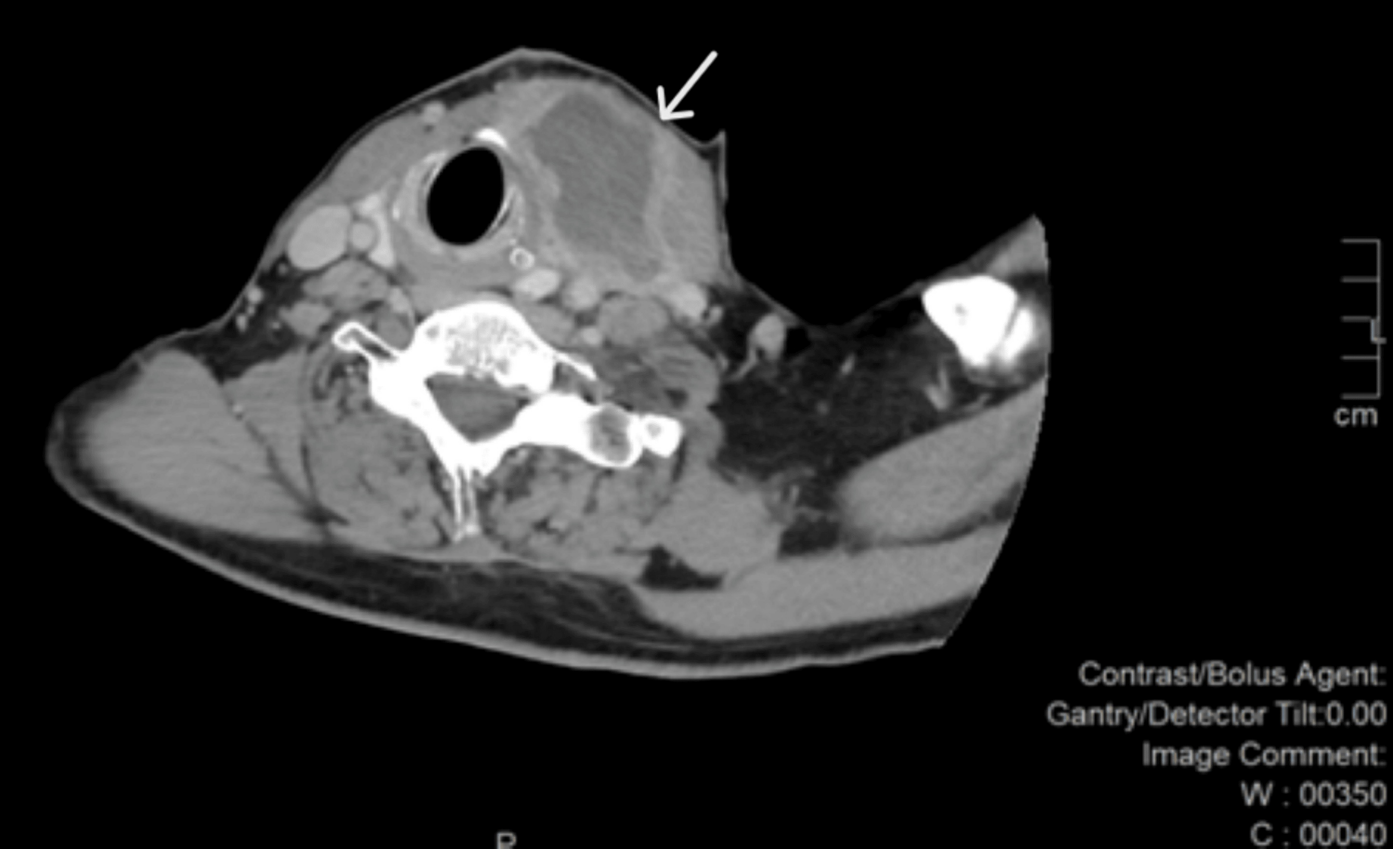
Neck CT - axial view: part of the tumor with cystic degeneration (white arrow).
